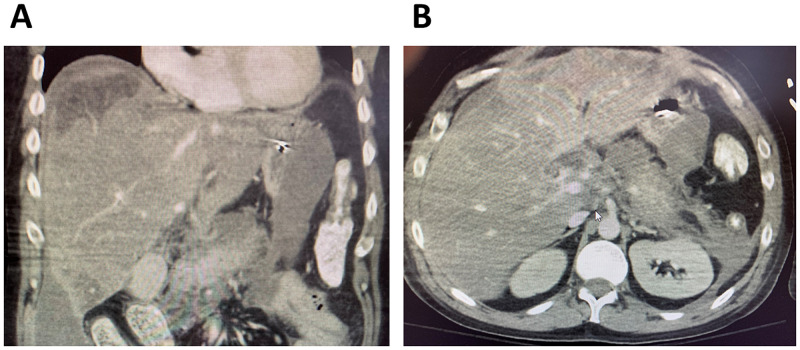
Abdominal CT demonstrating acute pancreatitis The patient presented with abdominal pain and elevated lipase, concerning for acute pancreatitis. An abdominal CT (A: sagittal, B: axial) scan was obtained, demonstrating extensive soft tissue and fat stranding about the pancreas and adjacent to the peritoneum, confirming the diagnosis. Given his extensive drinking history, a diagnosis of alcoholic pancreatitis was made.
